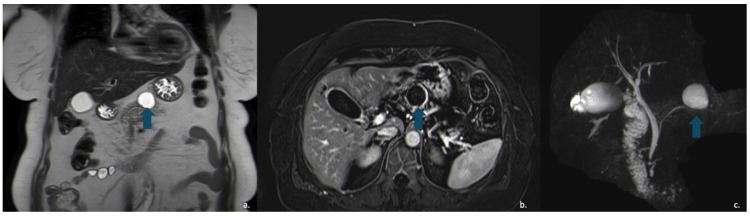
MRI of a 57-year-old man with neuroendocrine neoplasm (NEN) with cystic degeneration involving the pancreatic body (arrow). The lesion appears hyperintense on T2-w-HASTE sequences (a) and demonstrates peripheral rim-enhancement (b). MR cholangiography shows no communication with the main pancreatic duct (c).
